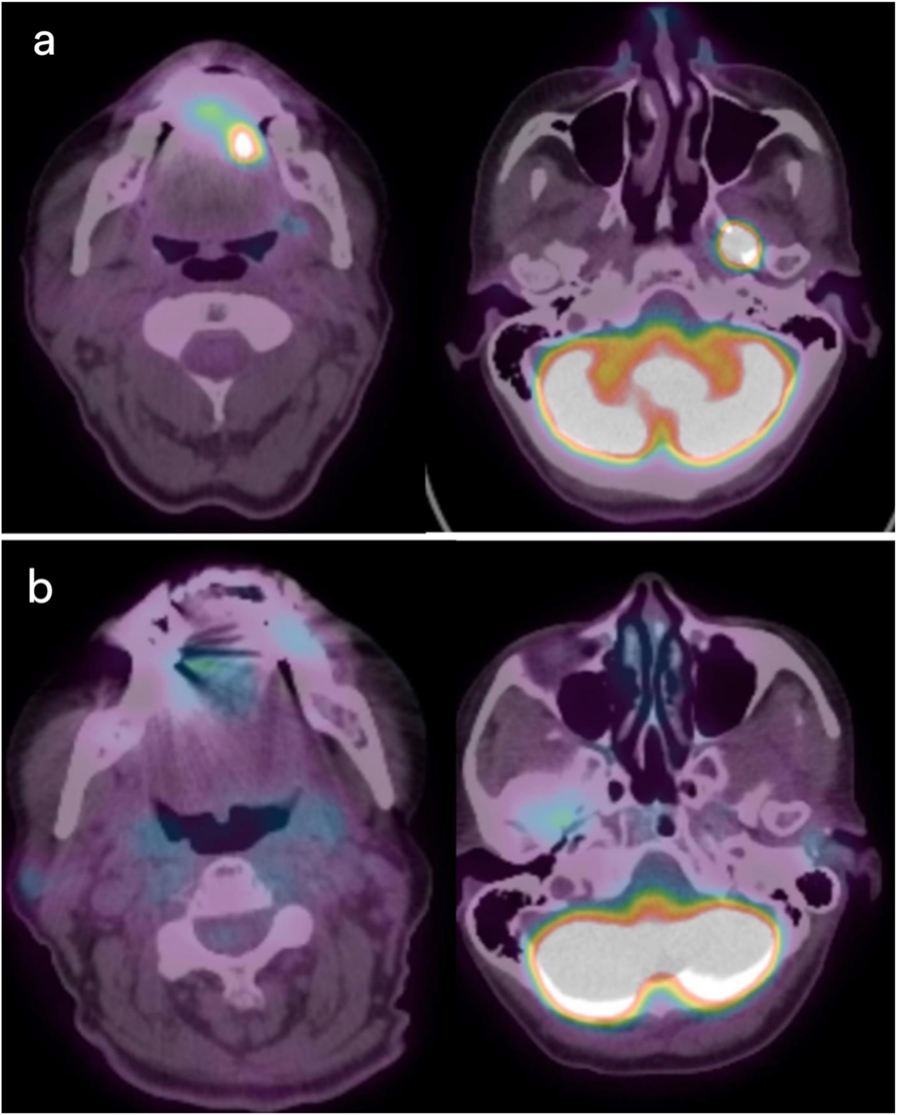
Representative fusion PET-CT images before and after R-CHOP chemotherapy. a Pre-treatment scans demonstrate intense uptake in the left oral tongue and left skull base. b Post-treatment scans demonstrate decreased uptake in previously intense areas
